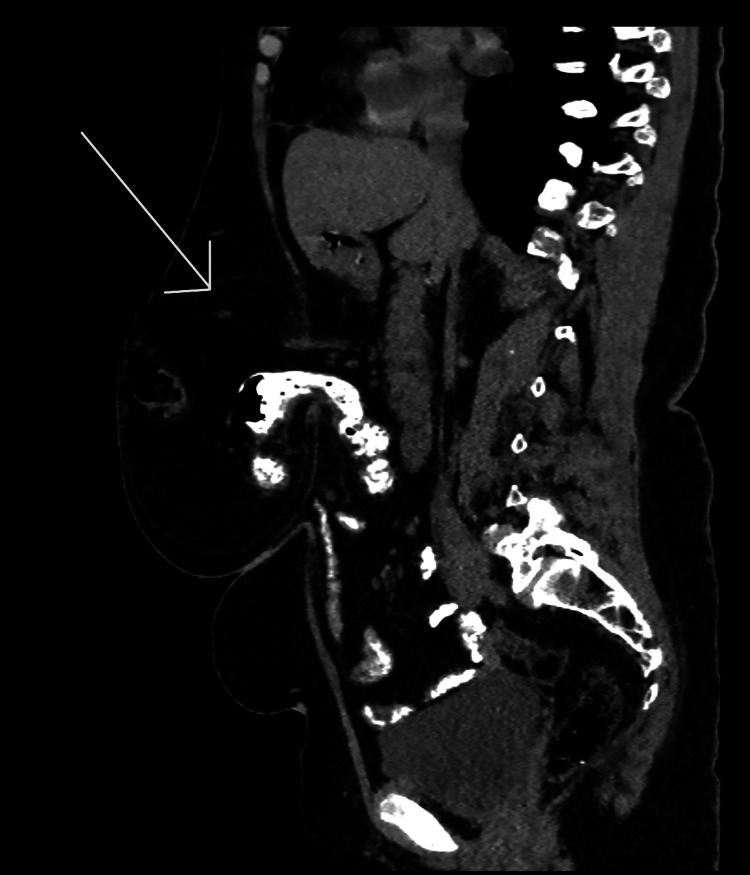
Sagittal view of a non-contrast CT scan with oral contrast showing bowel in the hernial sac (arrow)
